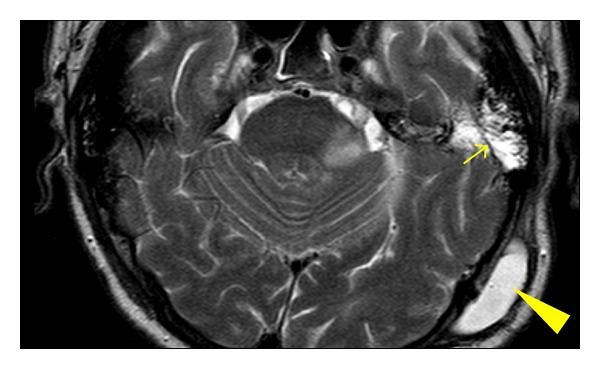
Lateral variant of CSF leak/pseudomeningocele (T2W MRI). Arrowhead: pseudomeningocele, arrow demonstrates CSF filled pneumatic system of the temporal bone.
